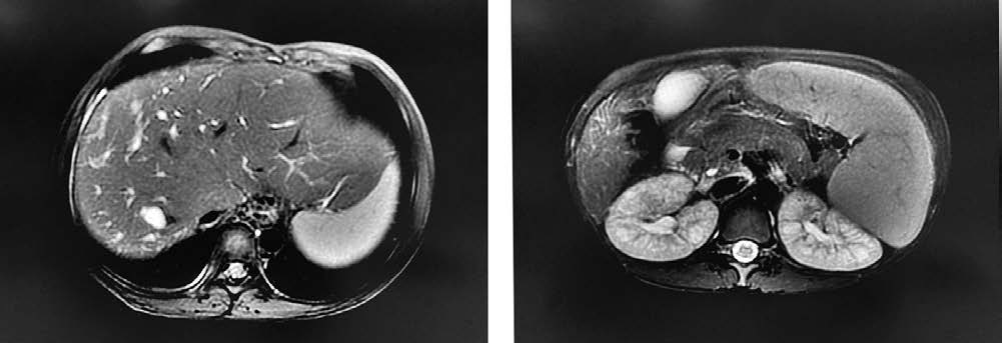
MRI suggested enlarged liver, dilated intrahepatic bile ducts with some distal cystic dilatation, dilated common bile ducts, splenomegaly, increased volume of both kidneys, and mildly dilated fluid accumulation in some of the calyces of both kidneys. MRI = magnetic resonance imaging.
